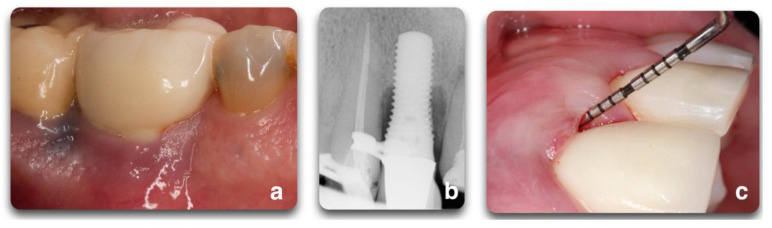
Representation of peri-implant clinical parameters that, associated, can lead to a diagnosis of peri-implantitis. In the sequence above, BOP/Suppuration (a), radiographic bone level ≥ 3 mm (b) in combination with PD ≥ 6 mm (c).
